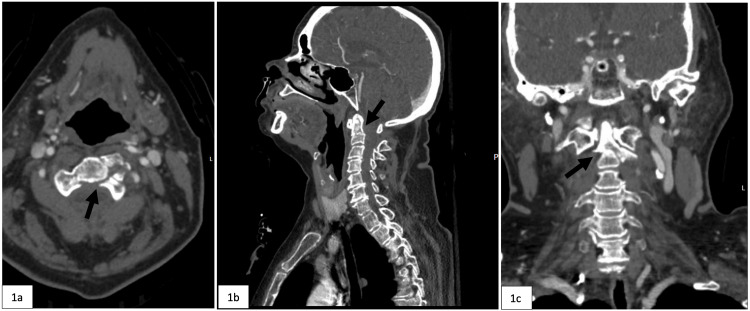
CT images demonstrating the type III dens fracture indicated by the black arrow on (a) axial view, (b) sagittal view, and (c) coronal view CT: Computed tomography
